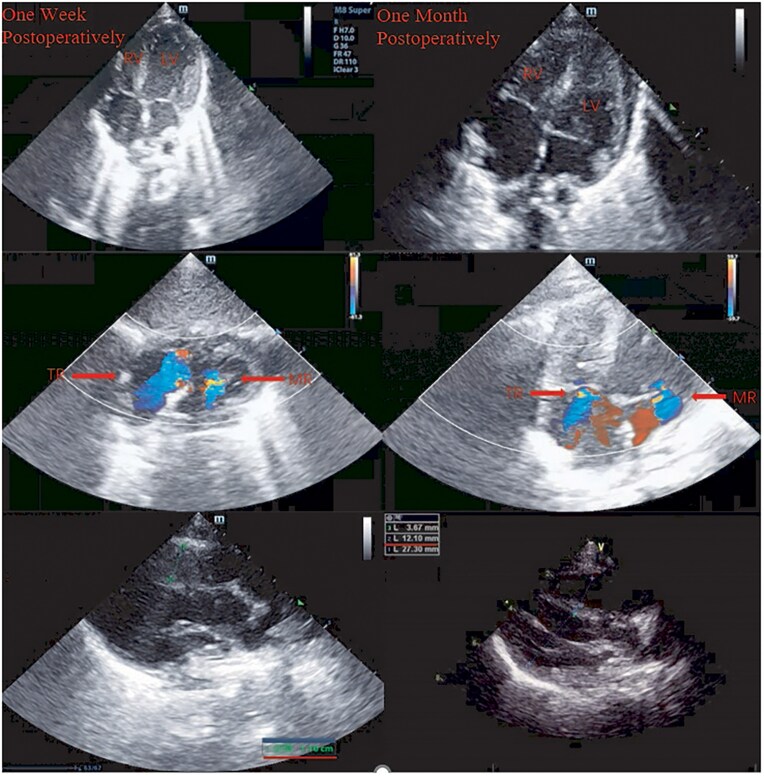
Comparison of echocardiography between 1 week and 1 month postoperatively. Note: LV = left ventricle; RV = right ventricle; TR = tricuspid regurgitation; MR = mitral regurgitation.
